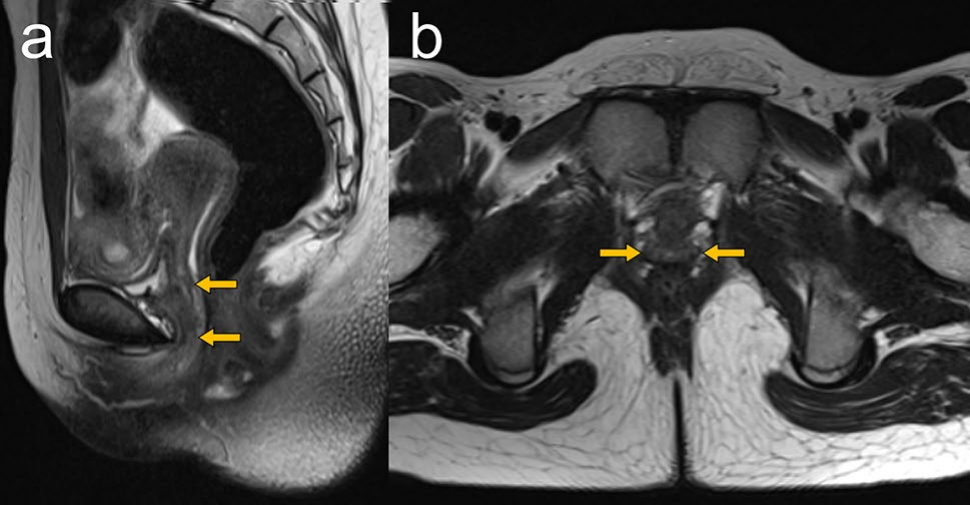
An 11-year-old girl after vaginoplasty for distal vaginal agenesis. Sagittal (a) and axial (b) T2-weighted MRI images show a neovagina (arrows). No postoperative complications, such as hydrometra due to vaginal stenosis or vaginal shortening, are observed
